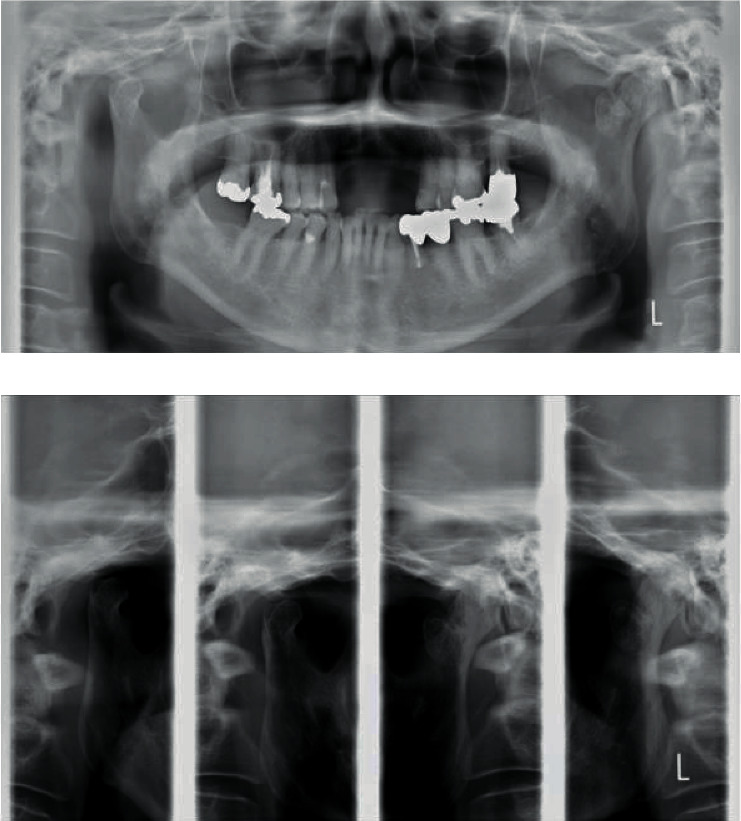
Radiographic findings: (a) panoramic radiograph and (b) lateral projection of the temporomandibular joint.
